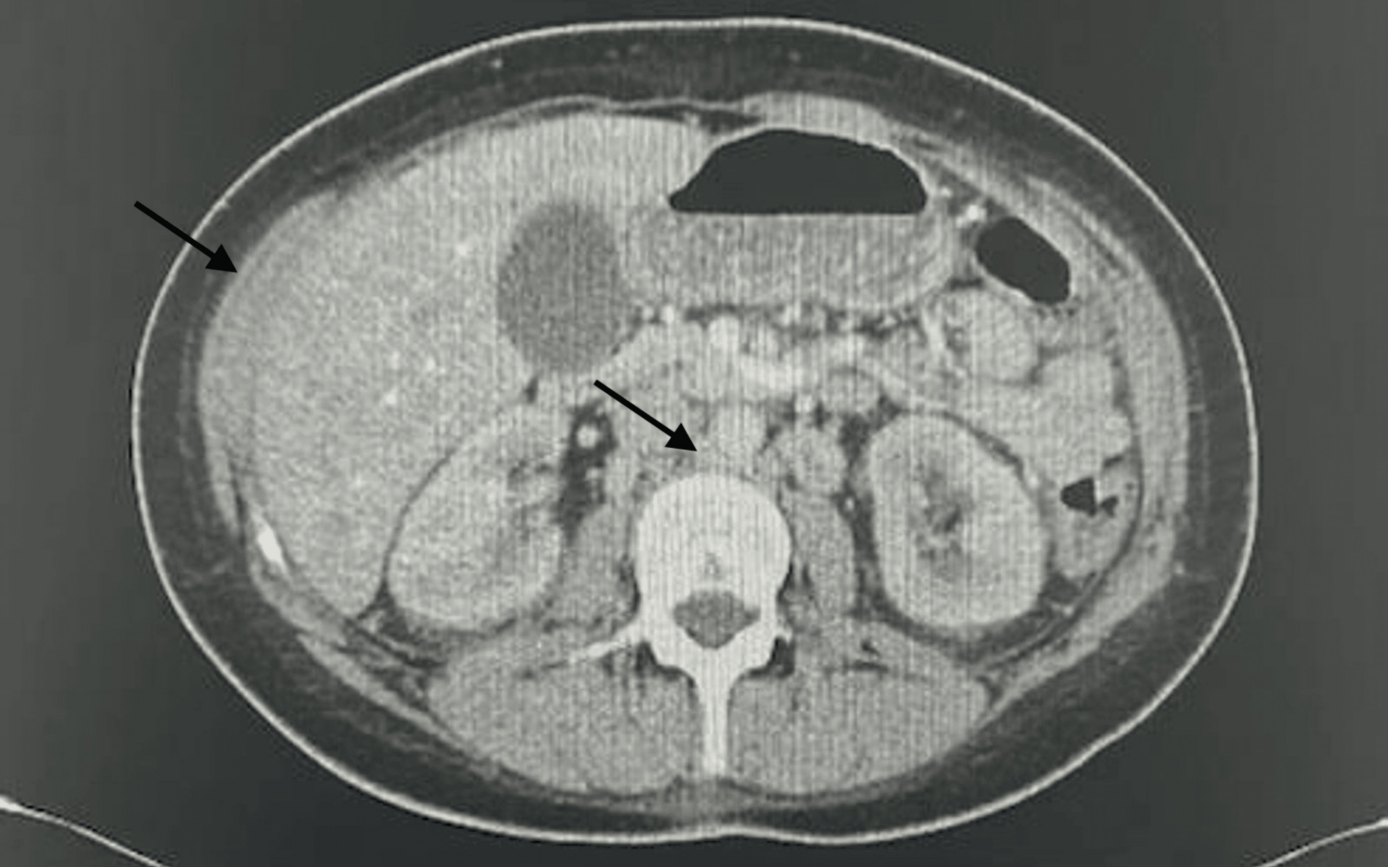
CT abdomen/pelvis Kidneys with normal enhancement, no hydronephrosis, similar left peri-aortic lymphadenopathy, new mild focal stranding in the right paracolic gutter, nonspecific.
